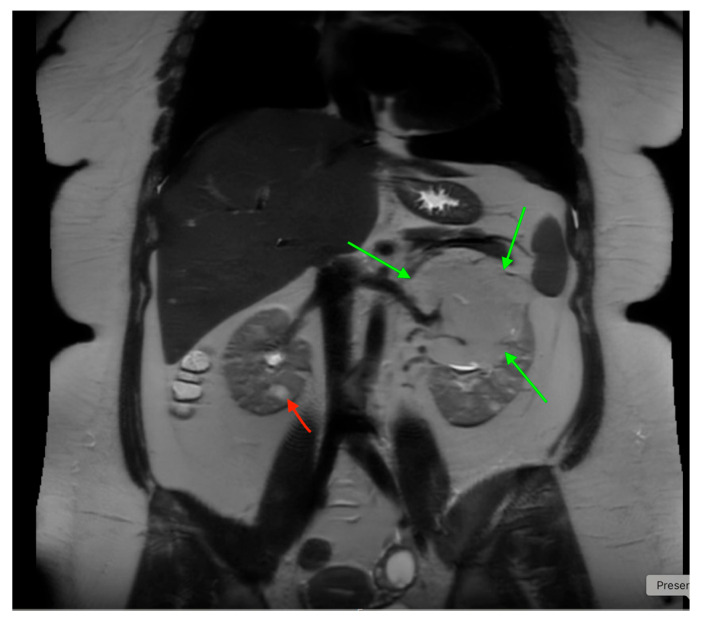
An abdominal MRI of an 18-year-old female with tuberous sclerosis reveals a large angiomyolipoma in the left kidney (marked by green arrows) and a smaller angiomyolipoma in the right kidney (marked by a red arrow).
